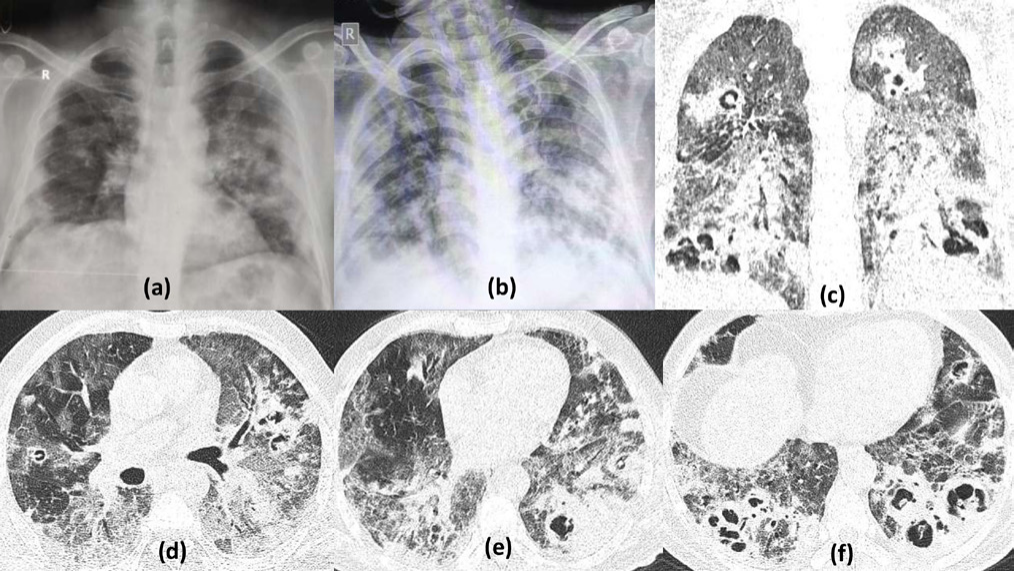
Multiple bilateral cavities due to severe COVID19 pneumonia: 60-year-old male with shortness of breath on high flow oxygen, positive for COVID19 infection. Frontal chest radiograph (a) on Day 6 of illness shows multifocal, fluffy, predominantly peripheral air space opacities in the right lower zone and left mid-lower zone. Serial chest radiograph (b) done on Day 20 (with worsened symptoms) shows progressive increase the infiltrates with a suspicious area of cavitation seen in the left mid zone. HRCT done few days later, axial and coronal sections (c–f) revealed multiple thick-walled cavities of varying sizes having irregular inner walls, within the areas of maximal consolidation bilaterally (predominantly in peripheral and lower lobe distribution typical of COVID19 pneumonitis)
